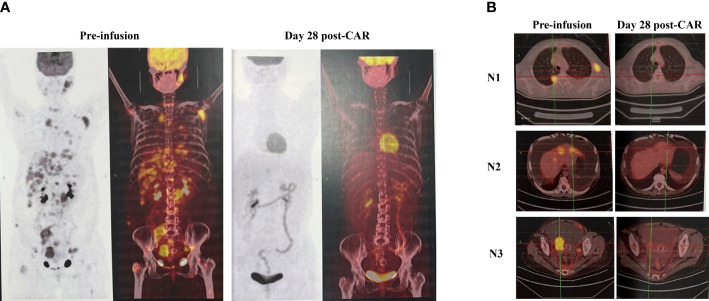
Clinical evaluation by PET-CT imaging. (A) Compared with the image before CAR T, PET-CT evaluation on day 28 showed nearly CMR with a few loci in the liver left. (B) The lymphoma activities were inhibited after CAR T cell infusion, as seen by PET-CT images for nodules at the lung, liver, and right adnexa. CMR, complete metabolic remission; N, nodule.
